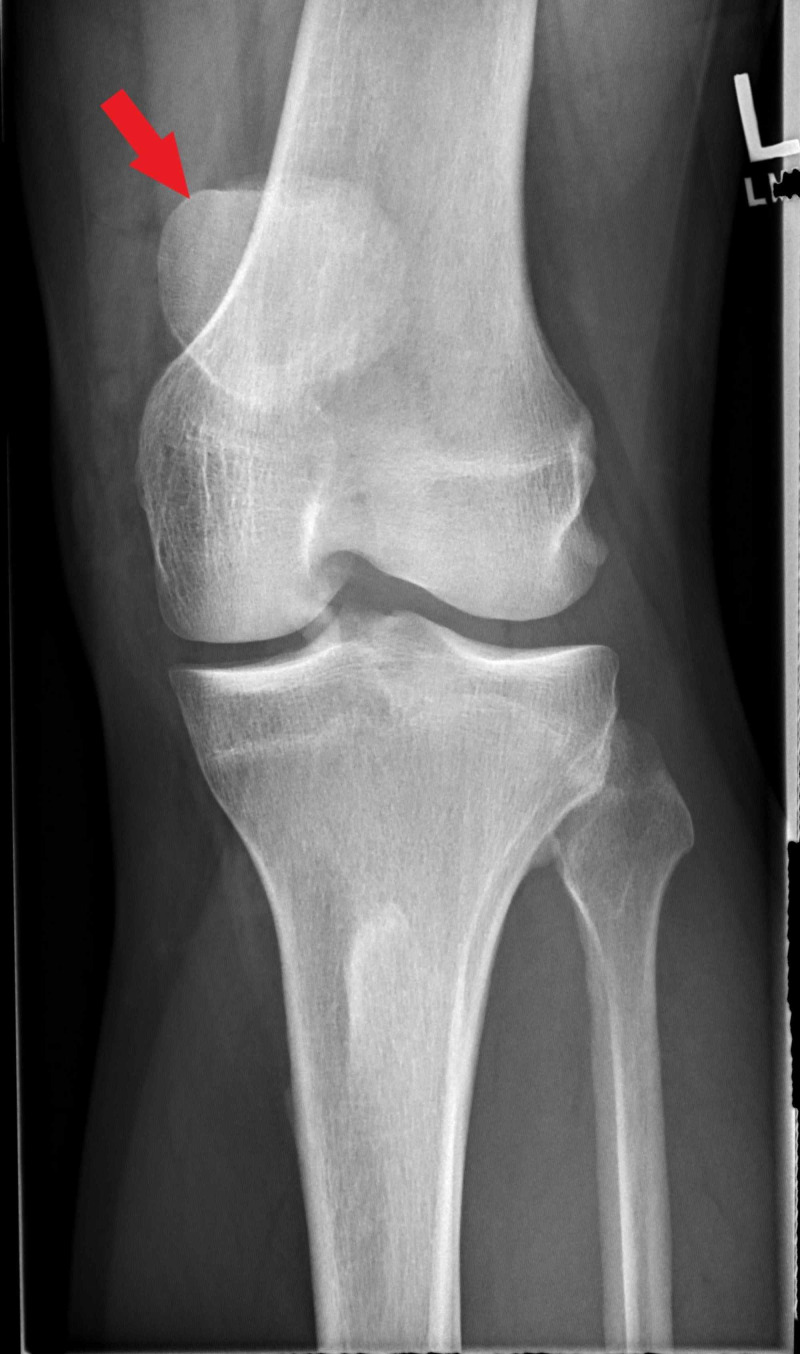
Anterior-posterior view radiograph of the left knee demonstrating high-riding patella (red arrow)
